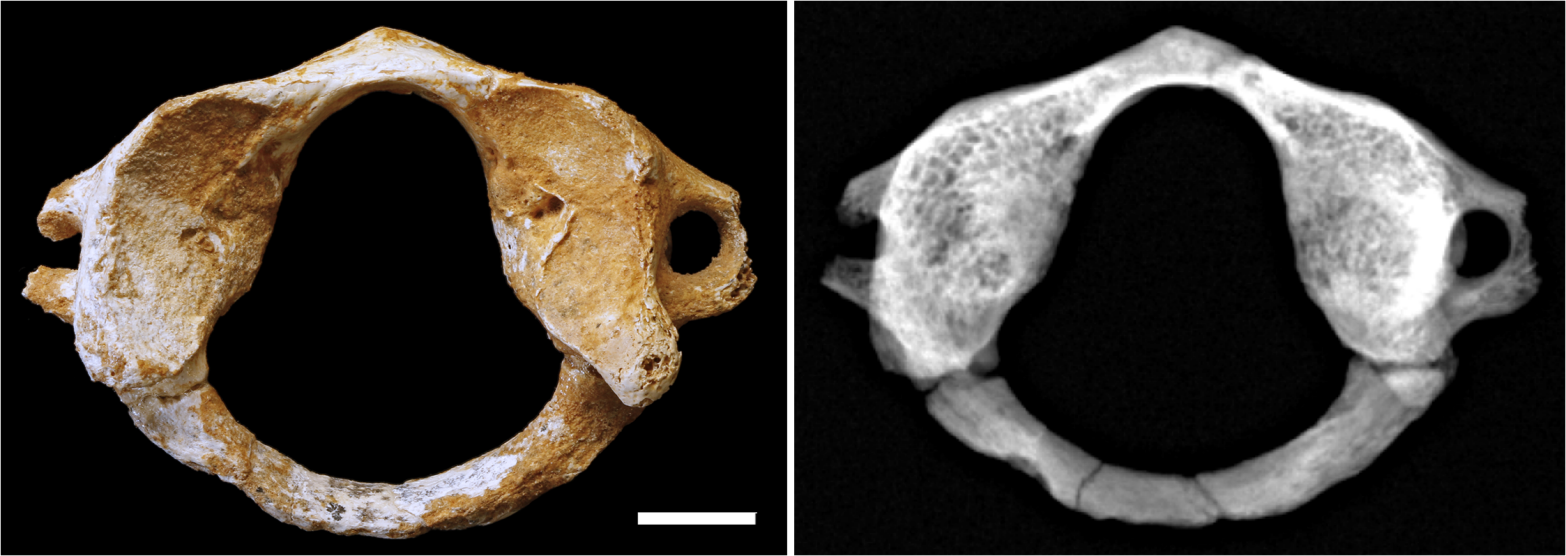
Atlas SD-1605/1595. (A) Superior view of SD-1605/1595, bar represents 1 cm. (B) Radiography of SD-1605/1595.
